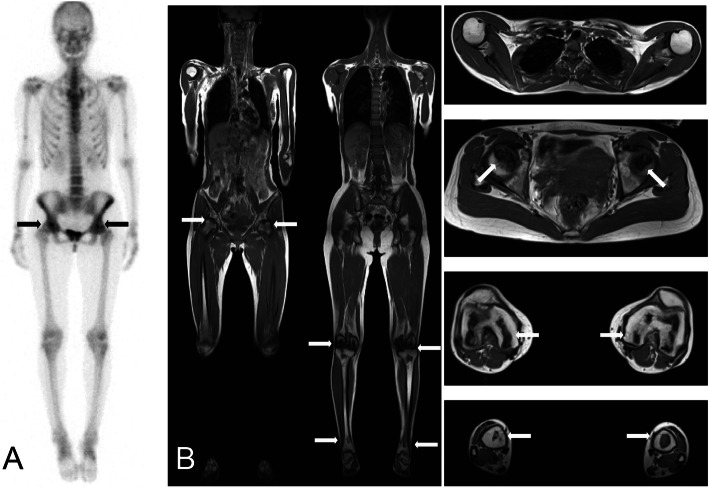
Osteonecrosis of the bilateral hip, knee, and ankle joints in a 38-year-old woman with a history of steroid use for scleroderma. a Coronal whole-body bone scintigraphy image. The arrows indicate the region of uptake in the bilateral hip joints. b Coronal and axial whole-body magnetic resonance image. The arrows indicate osteonecrotic regions in the bilateral hip, knee, and ankle joints
